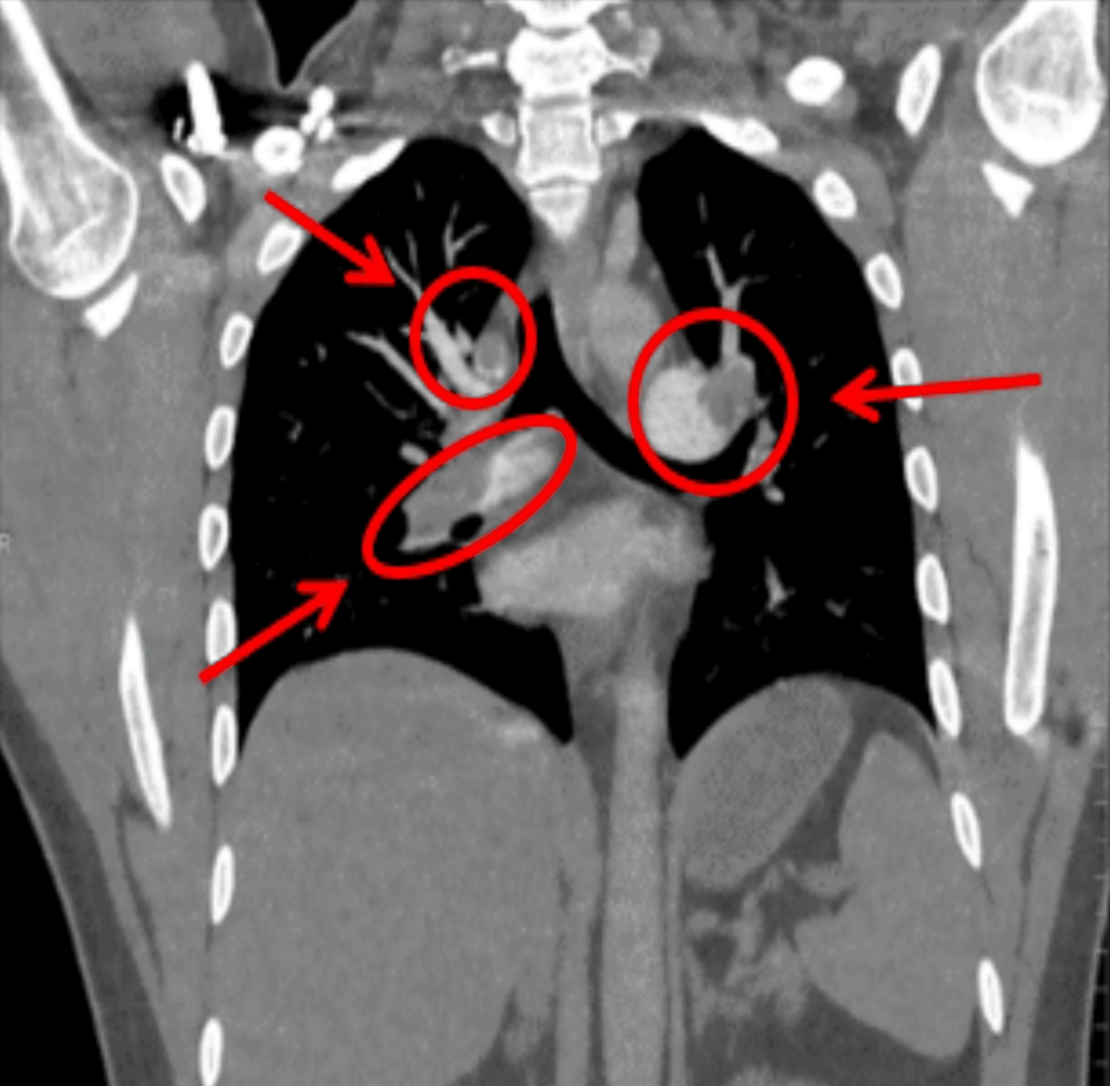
The CT pulmonary angiogram shows prominent filling defects in both the right and left pulmonary arteries, confirming the presence of bilateral pulmonary emboli The thrombi are within the central pulmonary arteries and extend into their proximal branches.
